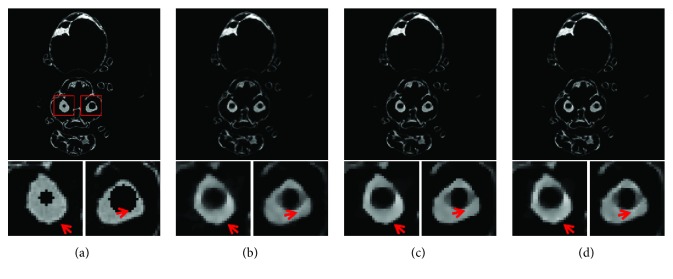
CT images of the modified bee reconstructed from inconsistent projections: (a) the reference image; (b) SART; (c) TV; (d) TF-L0. The second row images are the close-ups of ROIs. The places pointed out by red arrows are examples where TV and TF-L0 acquire different results.
